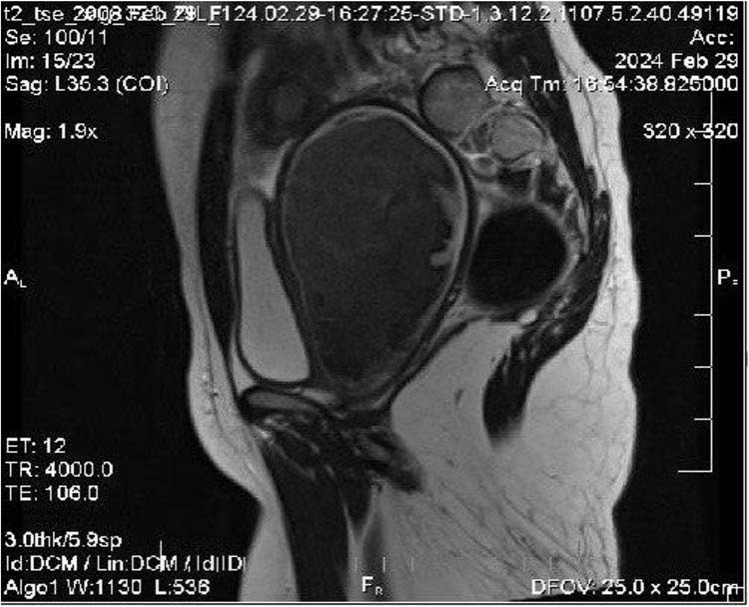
MRI imaging shows the level of vaginal obstruction.
